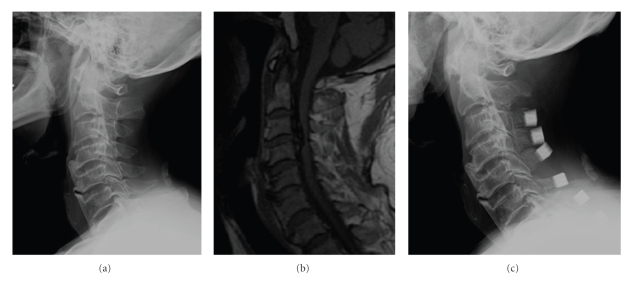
Preoperative image ((a): plain radiograph, lateral image, (b): MRI, sagittal image, and T1 weighted image). (a) Preoperative plain radiograph demonstrates significant multilevel cervical spondylosis. (b) In preoperative MRI, spinal cord compression at C3-4 due to OPLL is found. (c) Laminoplasty is performed using hydroxyapatite spacers from C3 to C7. MRI: Magnetic resonance imaging. OPLL: Ossification of posterior longitudinal ligament.
